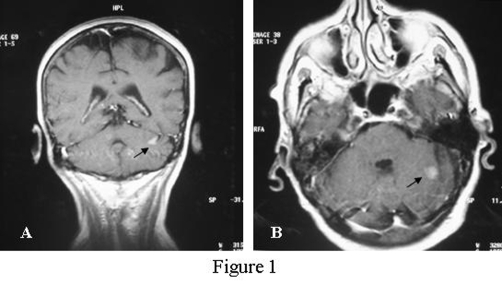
(A) Sagittal and (B). Axial MRI T1 weighted sequence where it is noted (black arrows) a metastatic lesion on the left side of cerebellum.
